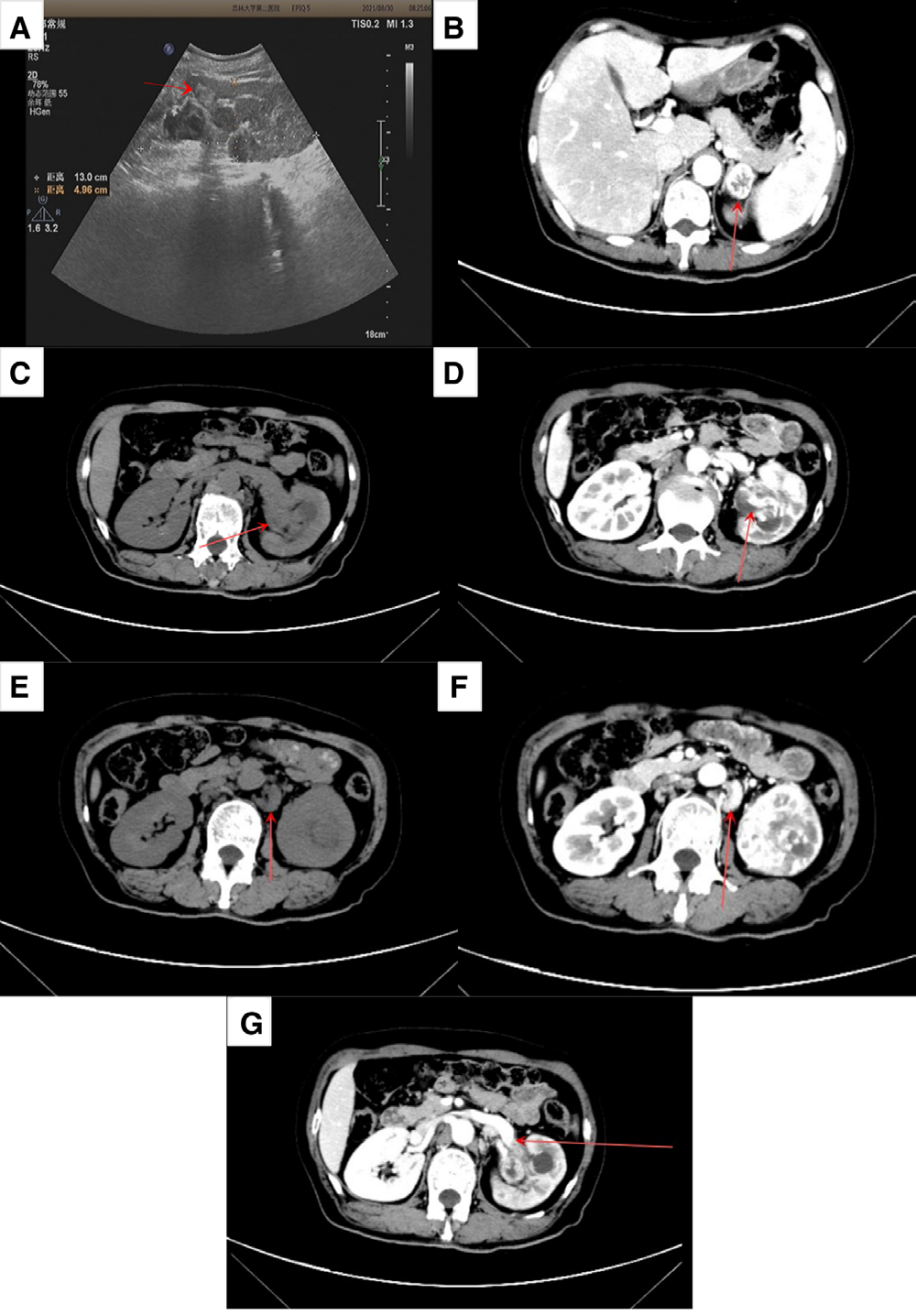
Renal pelvis carcinoma identified by ultrasound and CT scan. Ultrasound revealed the neoplasm in the left renal pelvis (A). Contrast-enhanced abdominal CT showed left adrenal involvement in the arterial phase (B), a tumor in the left renal pelvis in both the non-contrast phase (C) and arterial phase (D), and adjacent lymph node involvement in the non-contrast phase (E) and arterial phase (F). Additionally, a tumor thrombus was identified in the renal vein during the venous phase (G). The tumor is indicated by the red arrow. CT = computed tomography.
